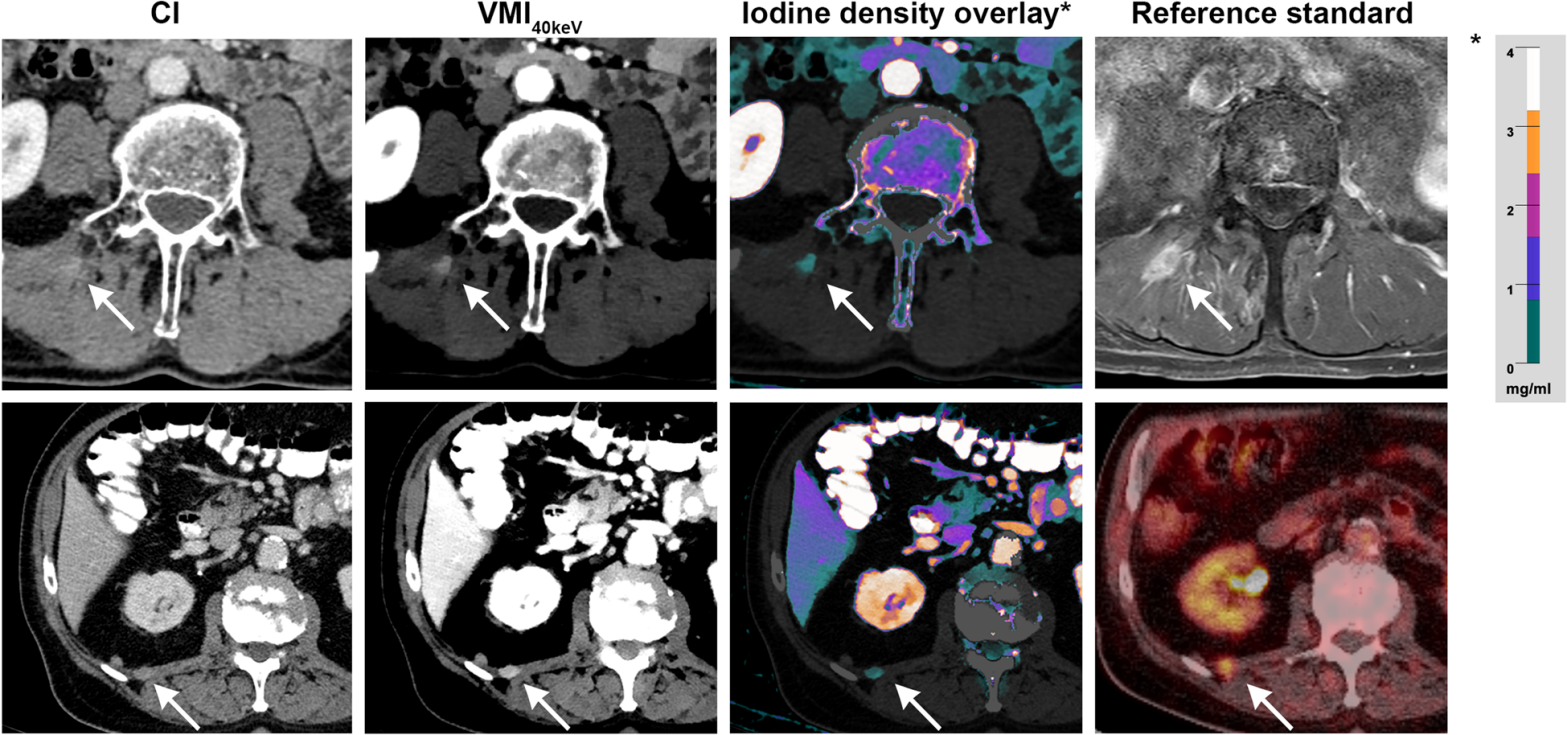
Exemplary cases of skeletal muscle metastases in CI, VMI_40keV_ and IDO with correlating MRI/PET-CT. Upper row: Metastasis of the right autochthone back muscle in a patient with malignant melanoma. Lower row: Patient with lung cancer diagnosed with a metastasis of the right quadratus lumborum muscle. Asterix indicates the color scale used in IDO
